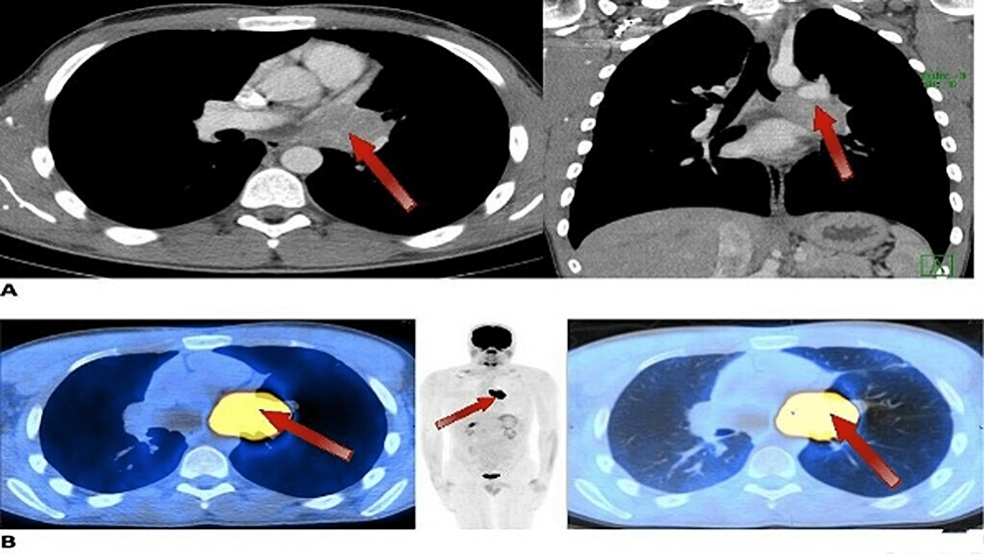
Pre-treatment imaging in January 2020 Axial and coronal CT images with contrast 1/21/20 (pane A) show a left hilar mass extending into the mediastinum measuring 4.3 cm x 3.1 cm that abuts the main and left pulmonary arteries, with an abrupt cutoff of the left upper and lower lobe central bronchi (indicated by red arrows). PET/CT fusion images (pane B) show the mass is intensely F-18 fluorodeoxyglucose (FDG)-avid (indicated by red arrows), with evidence of air trapping from the previously mentioned obstruction/cutoff of the bronchi.
